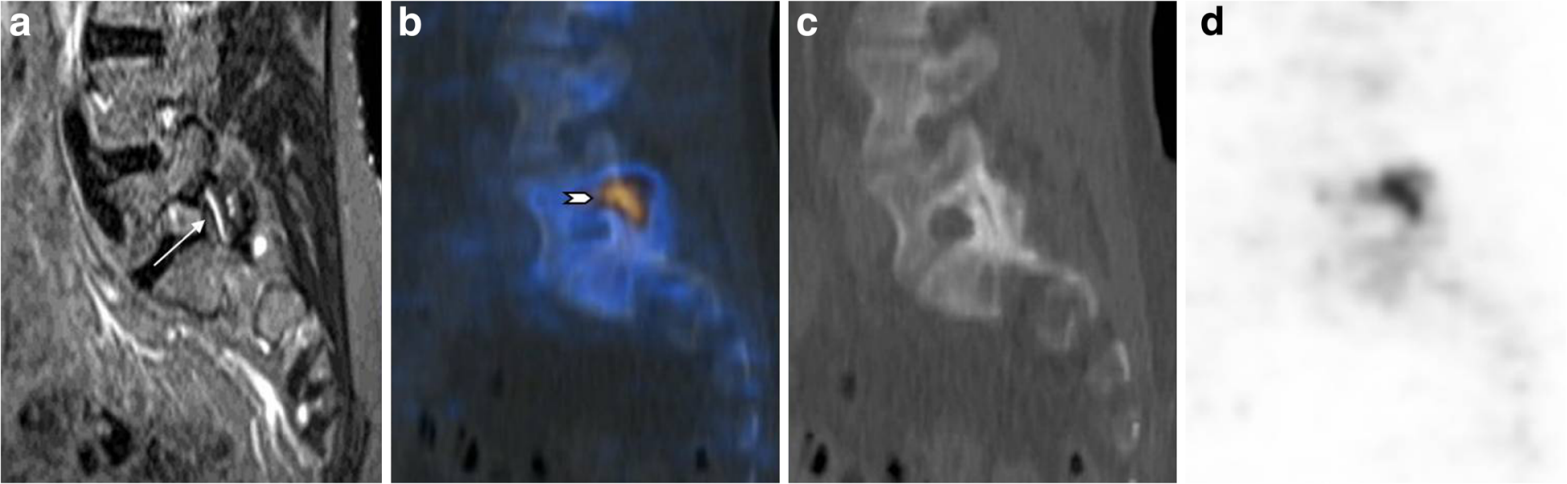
Fifty-five-year-old female patient (patient number 4). Patient with fluid within the left facet joint S1 (arrow) on MRI and corresponding increased activity on F PET/CT (arrowhead) indicating activated degenerative facet joint osteoarthritis. Left parasagittal STIR (a), fused PET/CT (b), CT (c), and PET (d) images.
